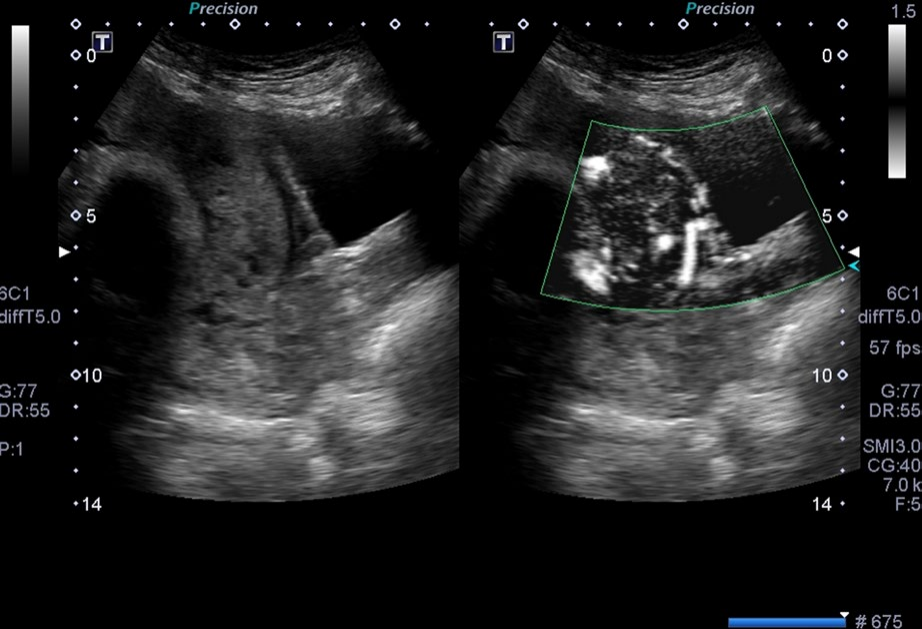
Sagittal transvaginal ultrasound Image shows abnormal irregular bladder uterine serosa boarder
